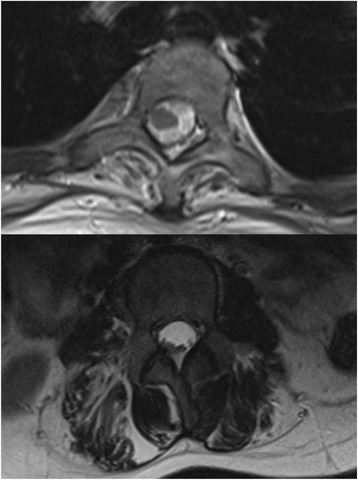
Magnetic resonance imaging (MRI) showed wasting and fat infiltration in paraspinal muscles
